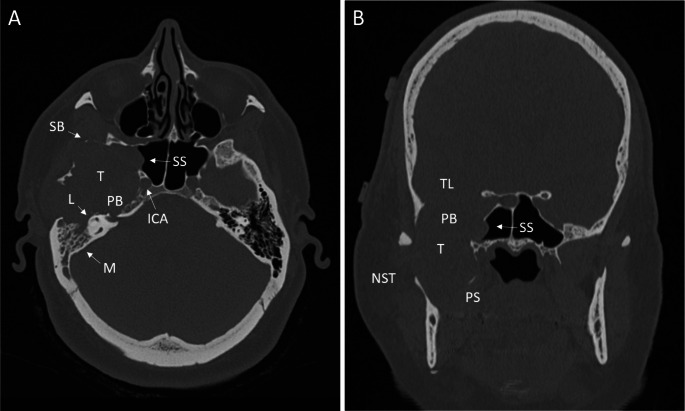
CT scan. A Axial and B coronar reconstruction. T: Tumor, SB: Destruction of the sphenoid bone, L: Labyrinth, PB: Destruction of the petrosal bone, M: Occluded mastoid, SS: Infiltration of the lateral wall of the sphenoid sinus, ICA: Internal carotid artery, PS: Infiltration of the parapharyngeal space, TL: Temporal lobe and invasion of the middle fossa, NST: Asymmetry of the neck soft tissue
